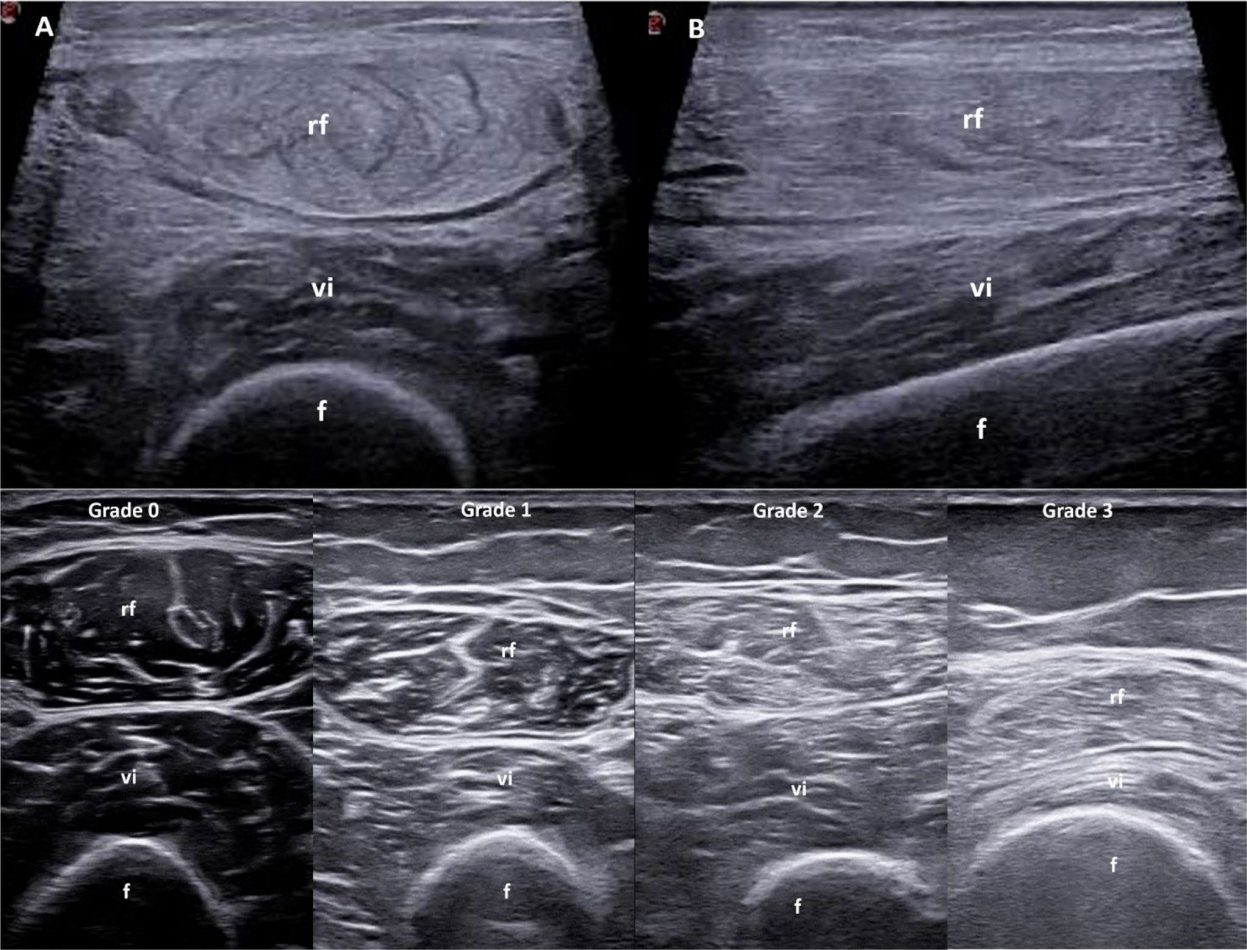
Ultrasound in muscle involvement (inflammatory myositis and sarcopenia). A shows a transverse scan, and B a longitudinal scan, both demonstrating widespread increased echogenicity without loss of the underlying bone signal (“see through” appearence) of the rectus femoris muscle with preserved muscle structure in a patient with polymyositis. Additionally, an example of a recently developed semi-quantitative scale for assessing muscle echogenicity (quadriceps muscle) in patients with sarcopenia is provided (reference no. 112). Images were obtained from patients with rheumatoid arthritis. *f* = femur; *rf* = rectus femoris muscle; *vi* = vastus intermedius muscle
